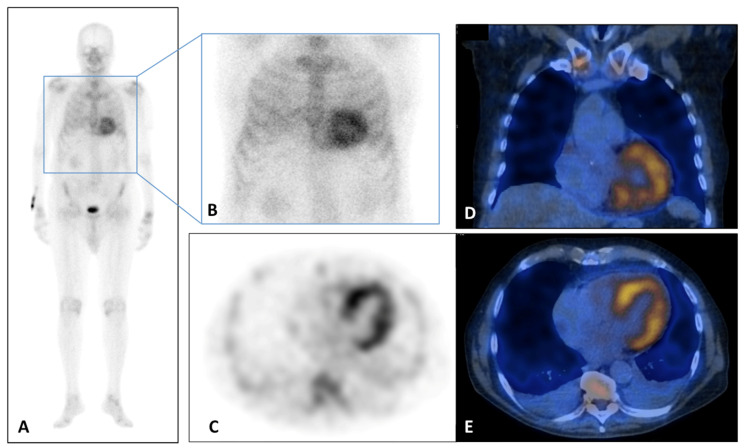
(A–E) 99Tc-Sn-HDP Scintigraphy performed in a 80 years old man with suspicious of cardiac amyloidosis and absent monoclonal protein. He presented to the emergency department for lipothymia and echocardiogram demonstrated signs of infiltrative cardiomyopathy with a LVEF of 40%, global longitudinal LV strain of −18% in absolute value. In addition, laboratory testing was significant for N-terminal pro b-type natriuretic peptide (NT-ProBNP) elevation at 5053 pg/mL (n.v. < 127.4 pg/mL). Whole Body (A) and static (B) planar bone scintigraphy, completed with tomographic imaging SPET (axial, (C)) and hybrid SPET/CT (coronal, (B) and axial, (C)) demonstrated moderate cardiac uptake. It correlated with score 2 of Perugini grading scale (cardiac uptake with intensity similar rib uptake), confirming the TTR amyloidosis diagnosis. LV, left ventricle; LVEF, left ventricular ejection fraction; NT-ProBNP, N-terminal pro b-type natriuretic peptide.
